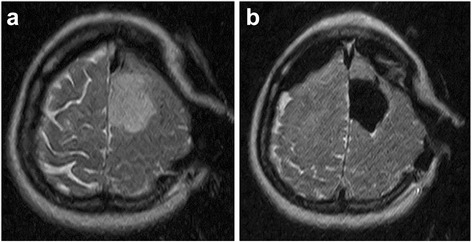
T2-weighted scout images of intraoperative magnetic resonance imaging scan. The first intraoperative magnetic resonance imaging was performed after craniotomy (a). Extent removal of the left frontal tumor was confirmed before surgical site closure (b)
